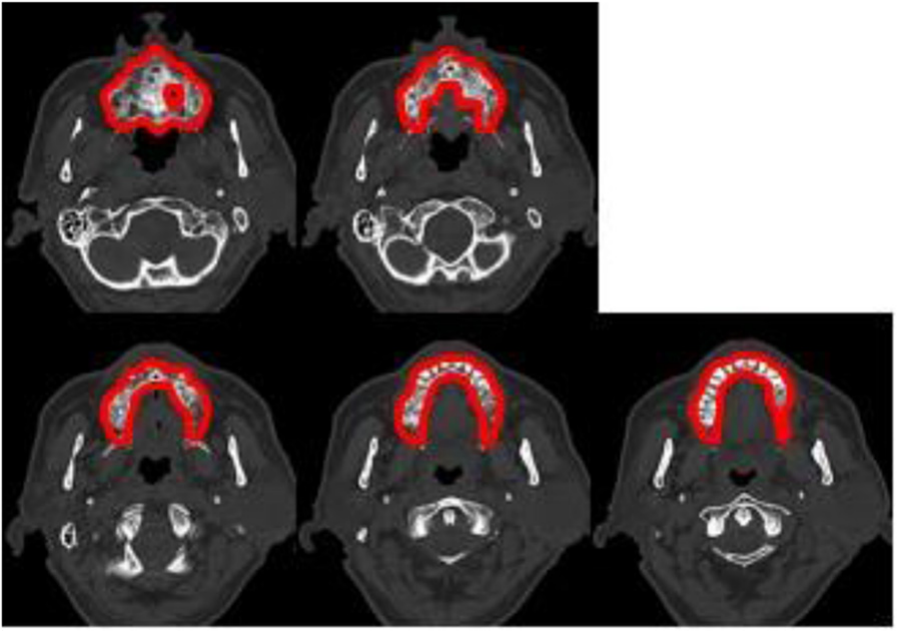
Representative contouring of the maxilla. All computed tomography images of the contoured maxilla are shown.
